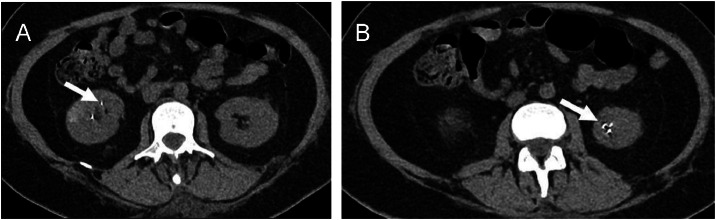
Abdominal axial CT scan in soft tissue window. (A) Unenhanced CT shows hyperdense foci in the inferior renal poles (arrows). (B) Enhanced CT revealed a relation of these foci with the renal arteries and some hypodense cortical wedge-shaped lesions corresponding to renal infarcts.
